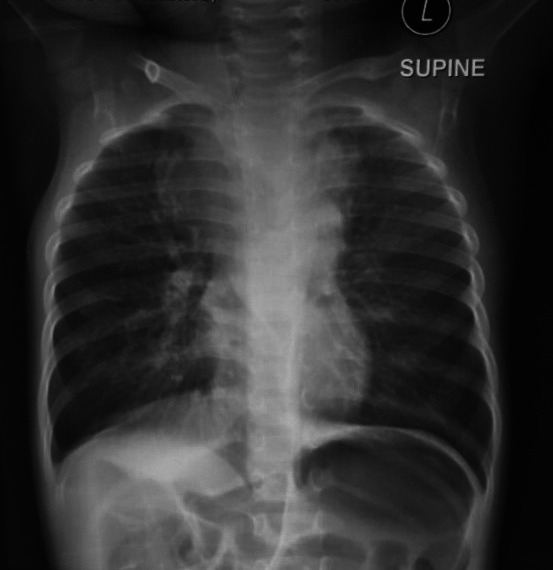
Pre-operative chest X-rays of the patient (not intubated).
